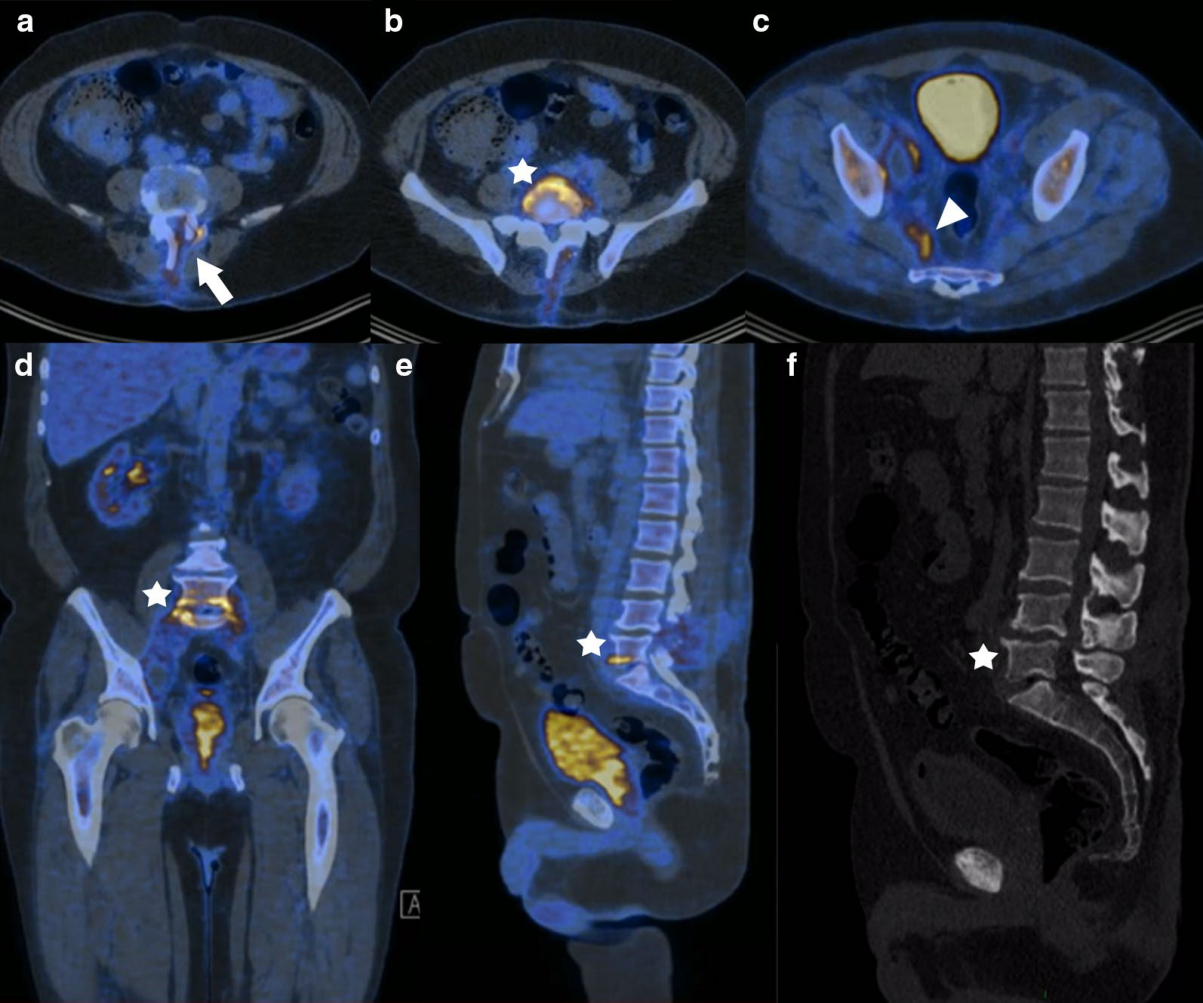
PET-CT in the setting of infection. PET-CT shows expected metabolic activity at the site of recent surgical intervention (laminectomy) (arrow) of the lumbar spine (a). Increased metabolism (b, d and e) associated with cortical irregularities and foci of osteolysis (f) are observed in the anterior aspect of L5 and S1 endplates (stars), consistent with ongoing inflammatory/infectious process. It is also observed a small fluid collection with increased metabolism in the right aspect of the pelvic cavity (c) (arrowhead)
